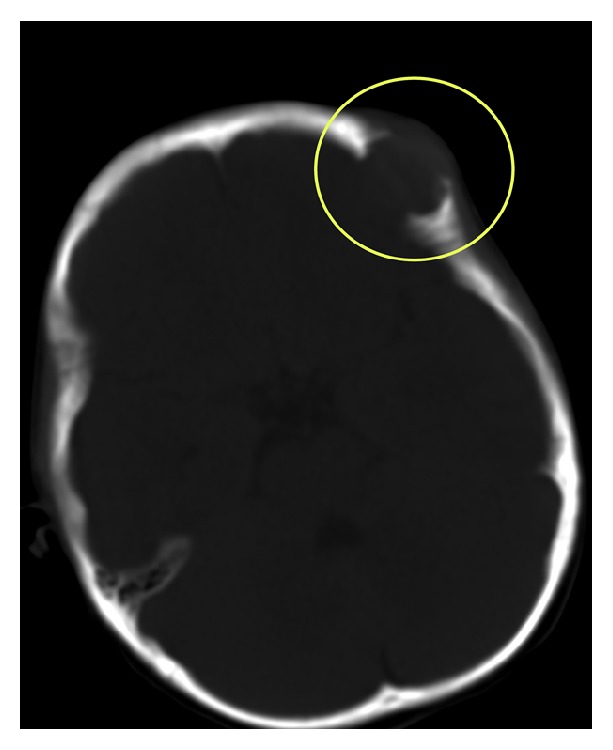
Images obtained from a 3-year-old female with IMT of the frontal bone. The CT scan showed destruction of the inner and outer plates, clear and sharp boundaries, and no calcification or ossification.
